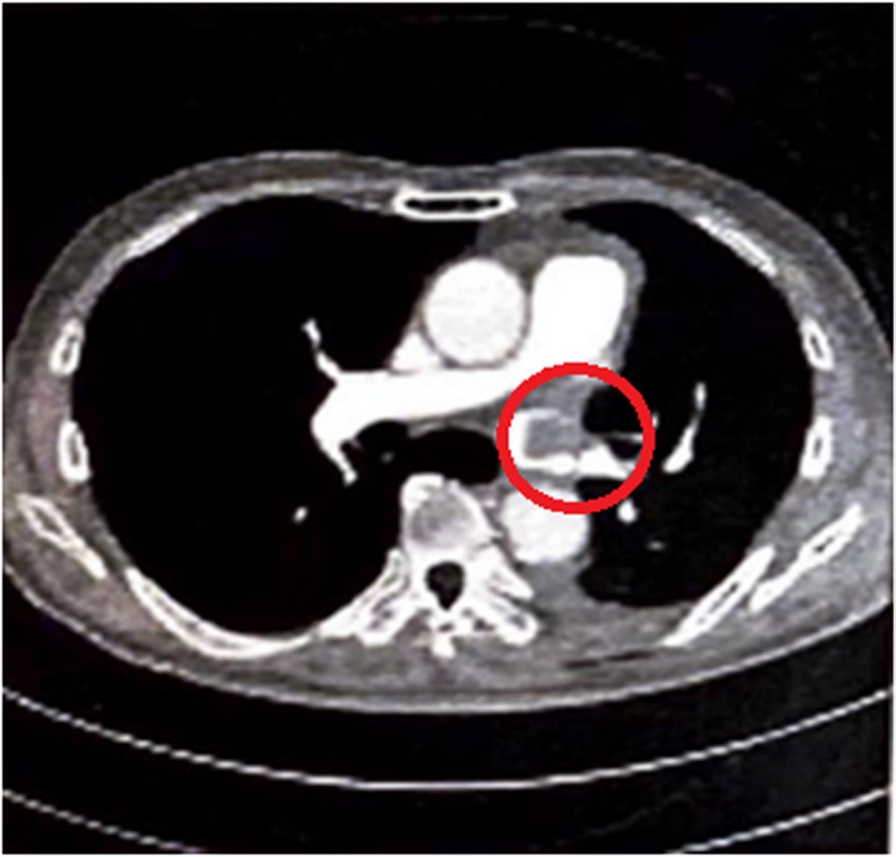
Contrast-enhanced chest computed tomography image. The circle indicates the pulmonary vein thrombosis
